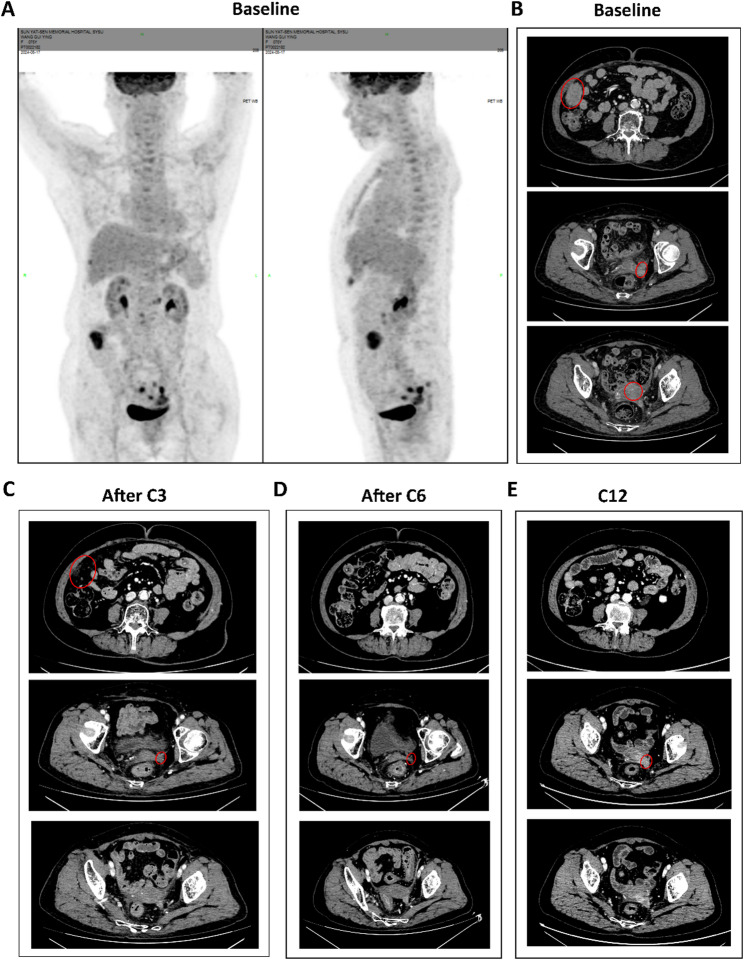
Imaging evaluation during the second-line treatment of the case according to Response Evaluation Criteria in Solid Tumors version 1.1. A PET-CT demonstrated multiple metabolically active lesions involving the whole body. B-E CT scans of target lesions from top to bottom: right abdomen, pelvis and cervix. B Baseline assessment. C Efficacy assessment after C3 (cycle 3) demonstrated a partial response. D Efficacy assessment after C6 (cycle 6) demonstrated a partial response. E Efficacy assessment on C12 (cycle 12) demonstrated disease progression with enlargement of the pelvic lesion
